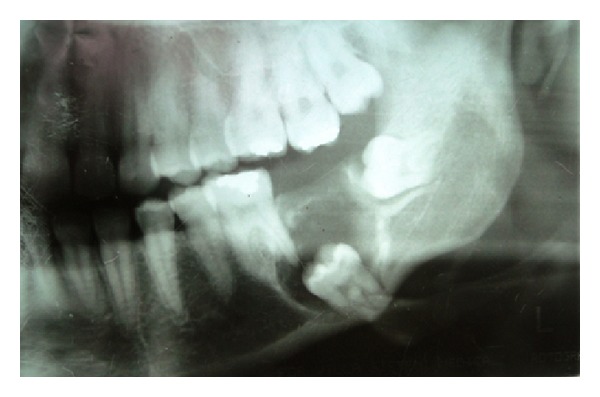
A panoramic radiograph showing a unilocular, radiolucent lesion, diffuse, associated with dental element 37.
